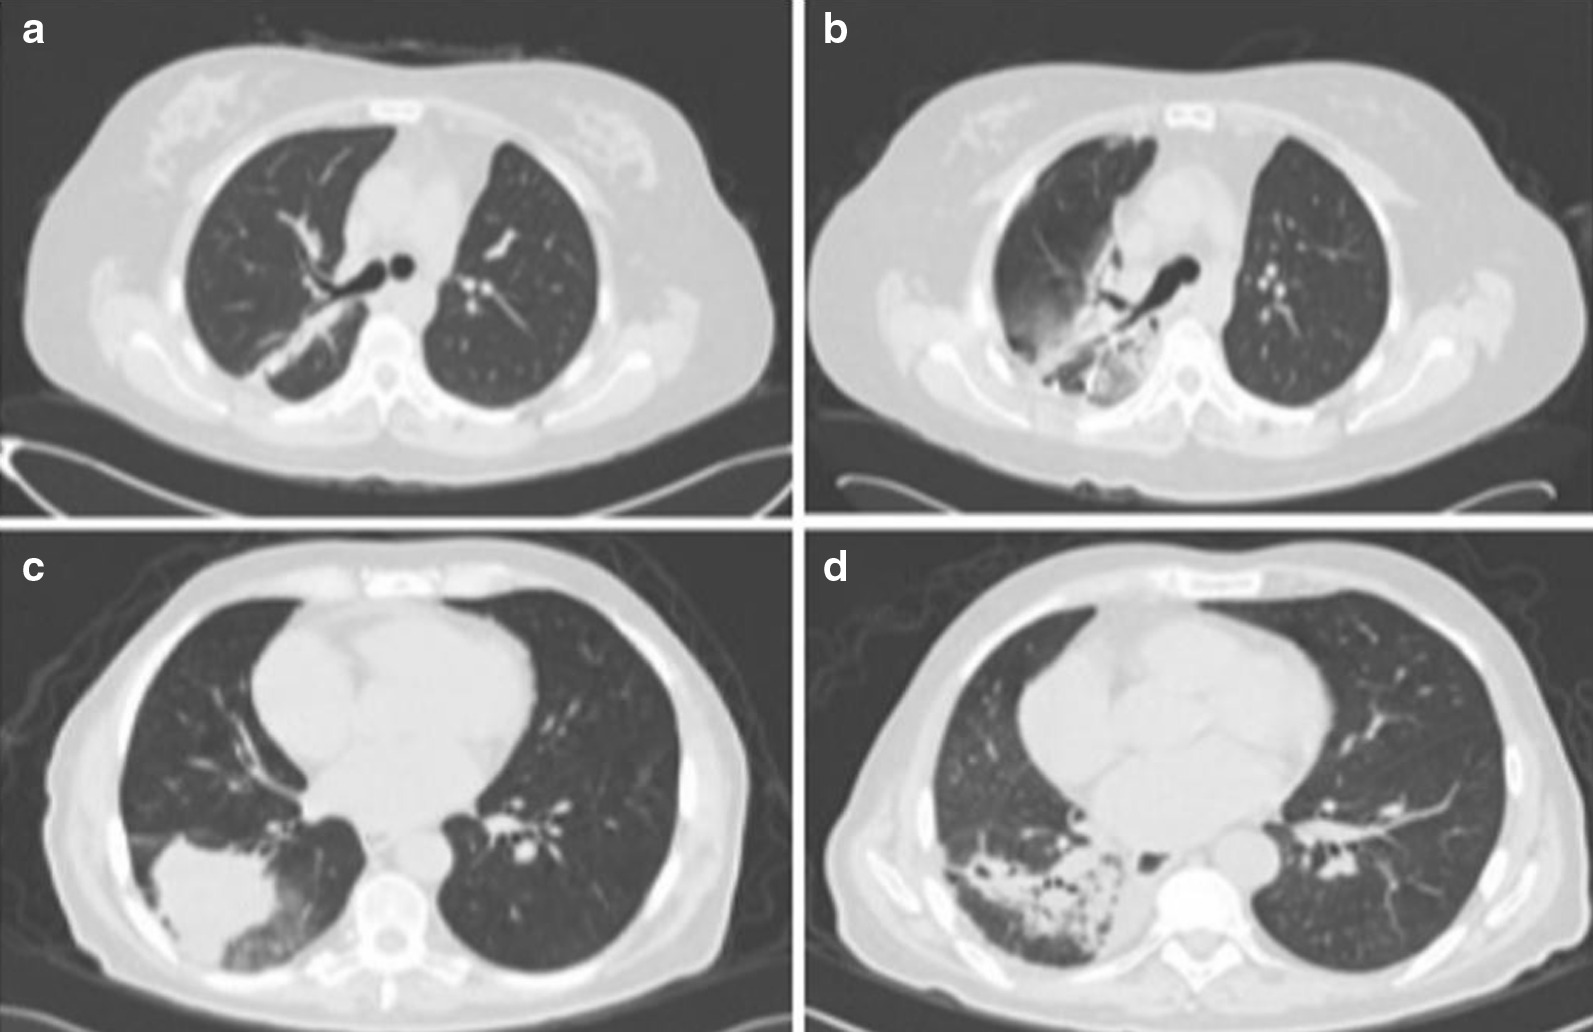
Imaging findings after radiation injury that can mimic other etiologies. a Axial images of a CT scan performed 1-week before RT and three months post-RT; b A ground-glass opacity image with atelectasis; c Axial images of a CT scan with tumor image in the lower lobe before RT and six months post-RT; d Consolidation, bronchiectasis, and a scar-like pattern.
